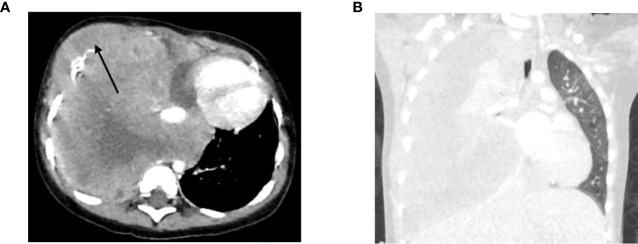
(A) Axial CT-scan (soft tissue setting) at diagnosis showing the primary tumor (indicated with an arrow) and (B) coronal CT-scan (lung setting) at diagnosis showing the pleural effusion.
